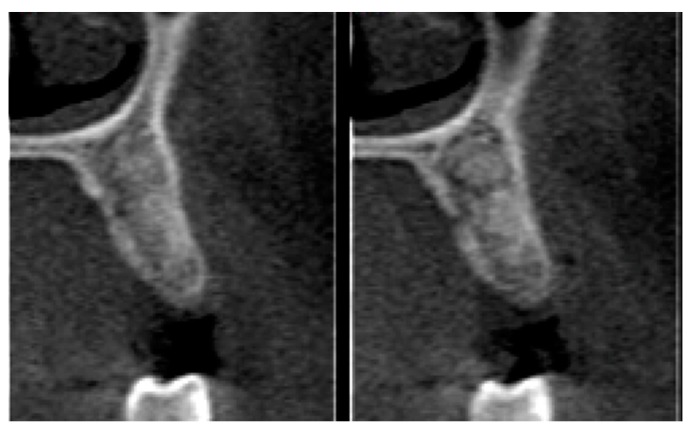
CT images of tooth 24 (TG2) 4 months after the procedure.
